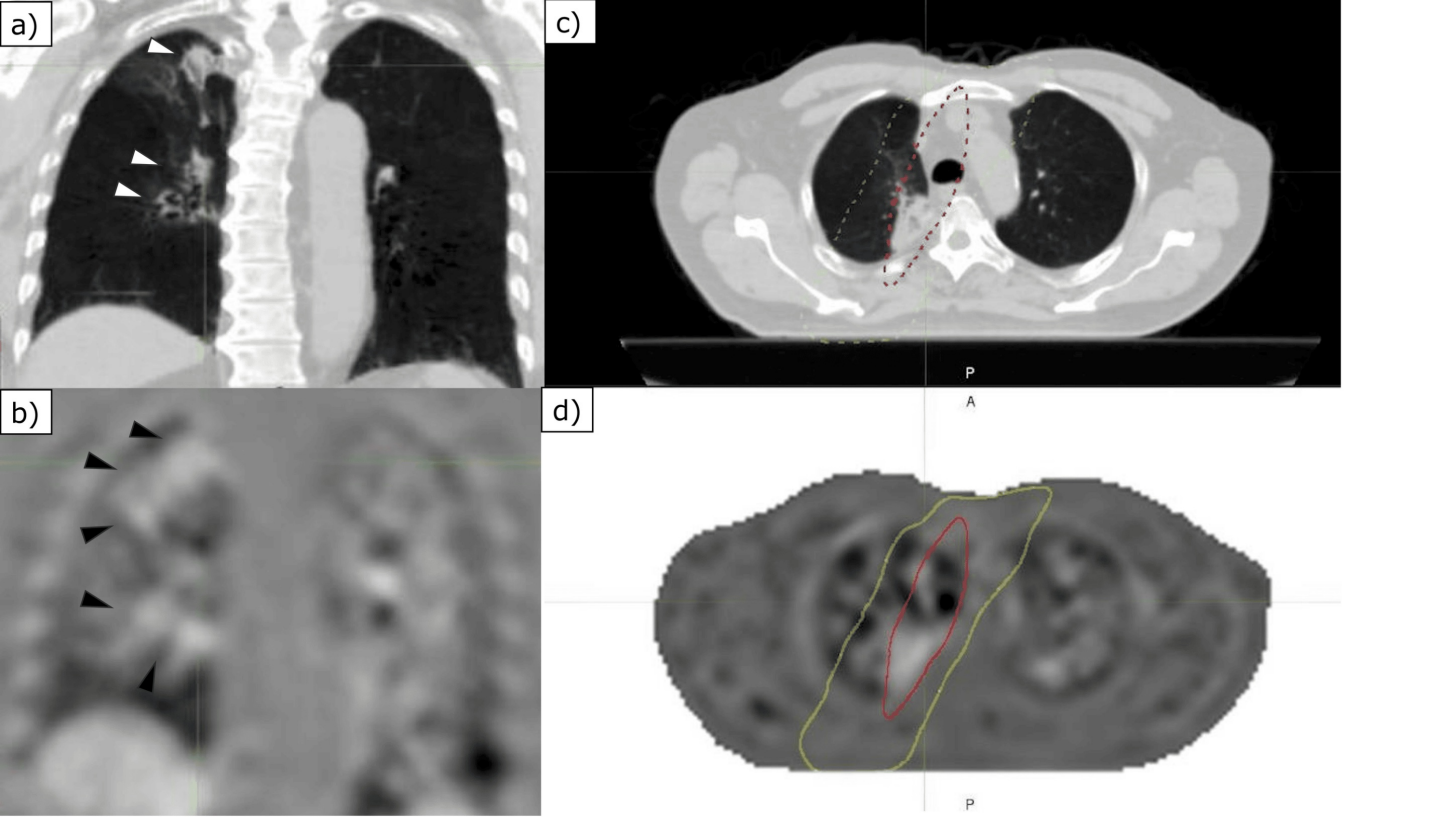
Comparison of four-dimensional computed tomography (4DCT) images and pulmonary ventilation images acquired one year post-treatment (a) Coronal images show the appearance of consolidation (white arrowhead) on the mediastinal side of the right lung in line with the irradiated field. (b) The corresponding lung ventilation image shows a wider range of hypoventilation than the consolidation seen in Panel (a). Dose distribution maps were superimposed on 4DCT and lung ventilation images to visually identify changes on the images and the percentage of the isodose line to which the hypoventilation site corresponded. (c) Axial image of the area where the primary tumor is located in the right upper lobe. The changes on the CT image correspond to the red-dotted 95% isodose line (62.7 Gy). (d) 4DCT ventilation image of the same slice as in Panel (c). The decrease in ventilation is noticeable at the red solid 95% isodose line, but it can also be visualized that it has spread to the yellow solid 30% isodose line (19.8 Gy).
